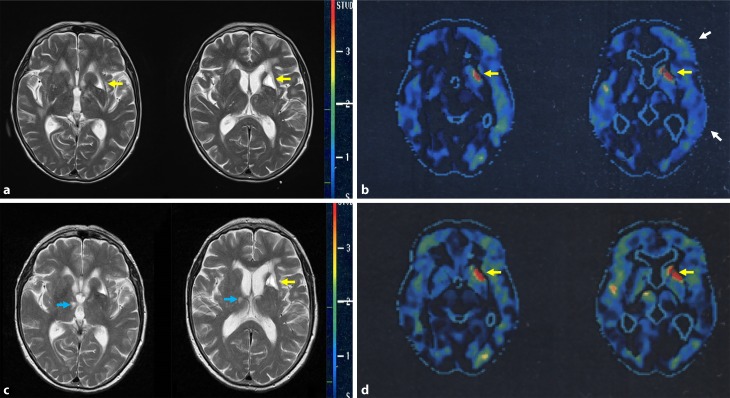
Patient 10, neuroimaging. MRI (T2-weighted images) 10 days before (a) and 9.5 months after antibiotic therapy (c). FDG-PET 2 months before (b) and 9.5 months after onset of antibiotic therapy (d). Metabolism evaluated by FDG-PET is presented as comparison with age matched healthy controls. Standard deviation is displayed with a red to dark blue scale. *White arrows* show hypometabolism in the left frontotemporal region before therapy (b) which is absent after therapy (d). *Yellow arrows* show left striatal lacunar lesion (a and b), not reversible after therapy (c and d). *Blue arrow* show small right thalamic vascular lesion (c), not seen in (a)
